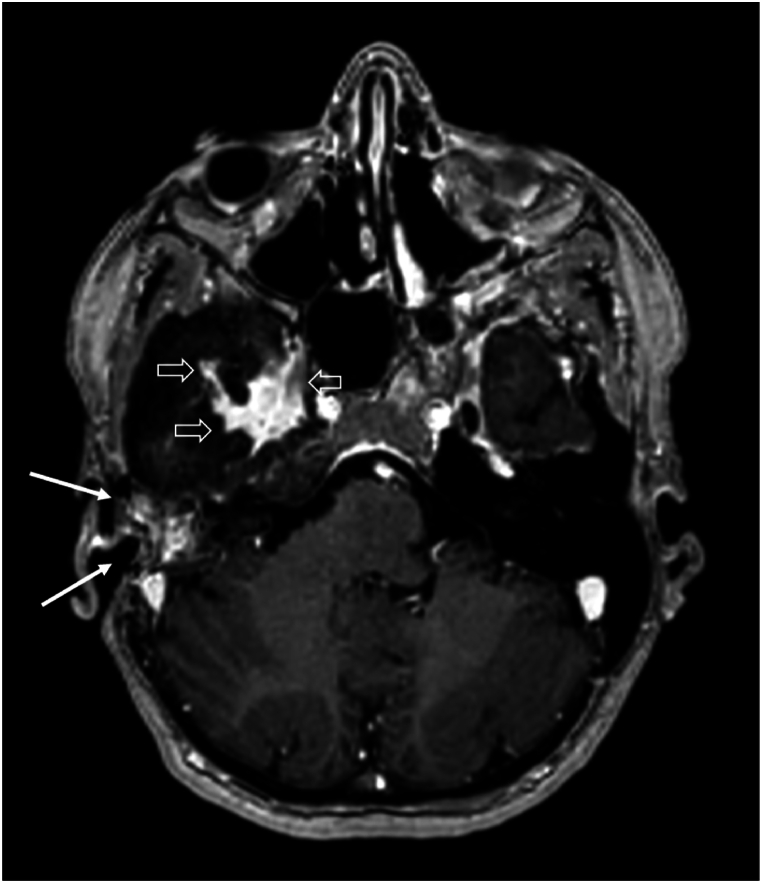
T1-weighted axial reconstruction after gadolinium. Hyperintense (fatty) signal after subtotal petrosectomy (white arrows). Hyperostosis of the sphenoid bone (hypo-intens signal) with solid enhancement at the middle posterior fossa, consistent with residual meningioma (open arrows).
